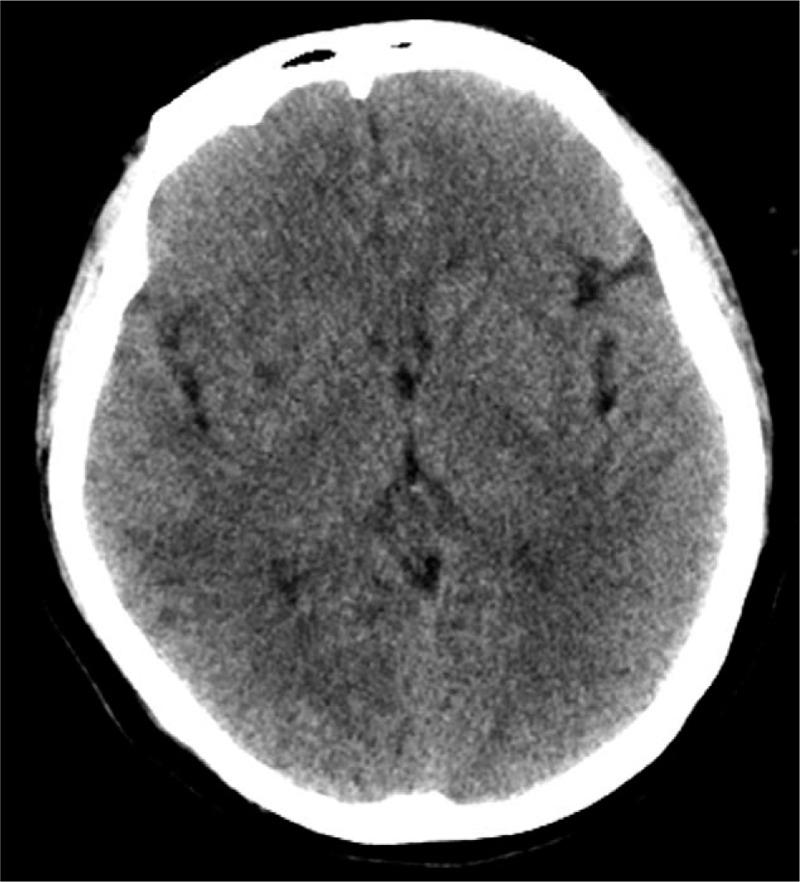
Follow-up CT scan taken 2 d later showing a hypodense lesion in the right hemisphere. CT = computed tomography.
